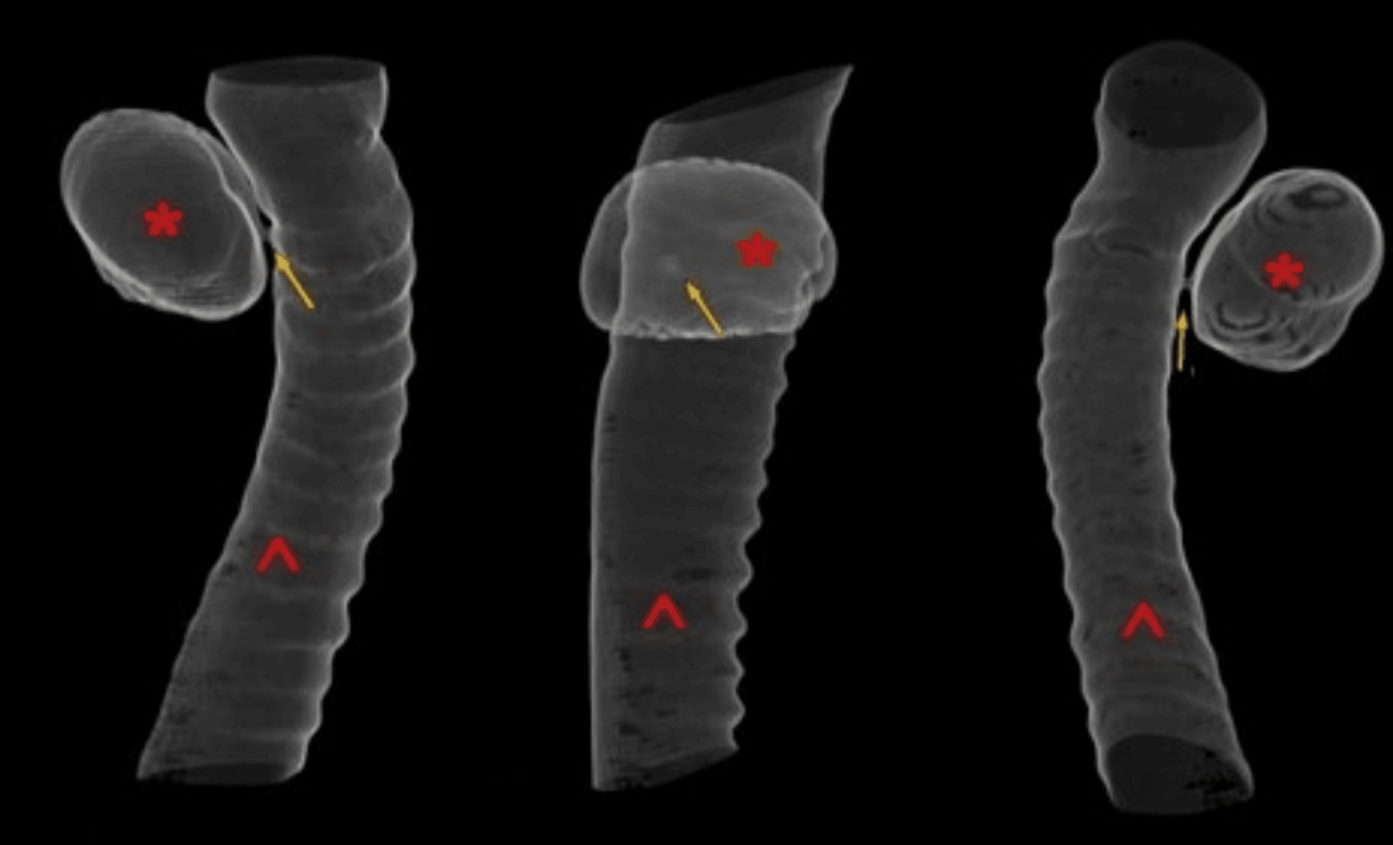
Three-dimensional reconstruction of the trachea derived from second CT scan (1.25-mm slice) during Valsalva maneuver Right-sided tracheal diverticulum (*), with detectable connection (yellow arrow) to the trachea (^)
